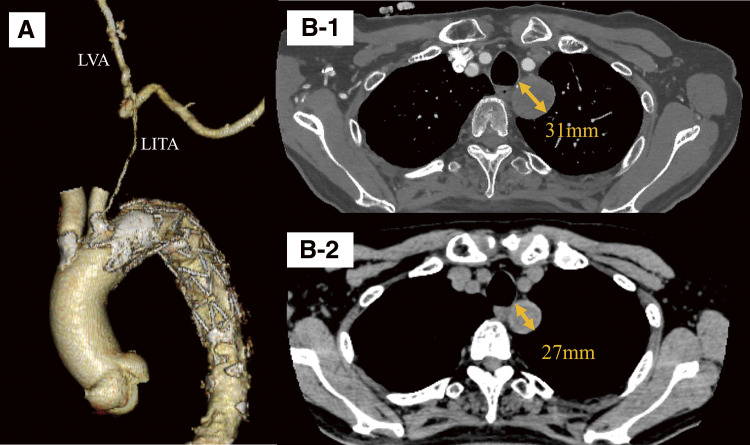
Fig. 3 (A) Three-dimensional reconstruction image of the immediate postoperative computed tomography. The left vertebral artery and left internal thoracic artery are preserved. (B-1) Axial image from the immediate postoperative period. No obvious endoleak is observed. (B-2) Axial image at 3 months postoperatively. The aneurysm has shrunk to 27 mm. LVA: left vertebral artery; LITA: left internal thoracic artery
